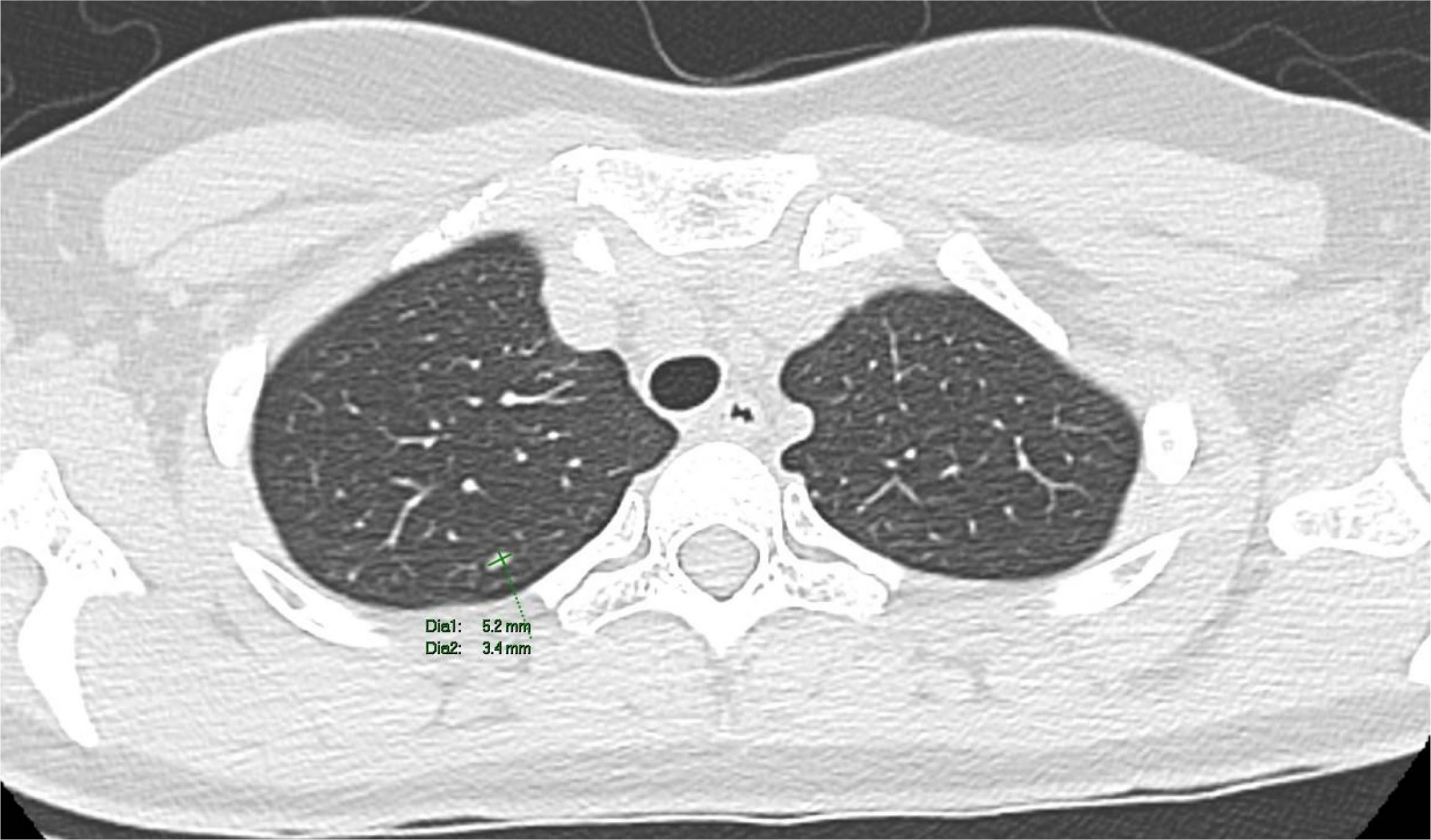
A computed tomography scan revealed non-calcified nodules in the upper lobe of the right lung.
